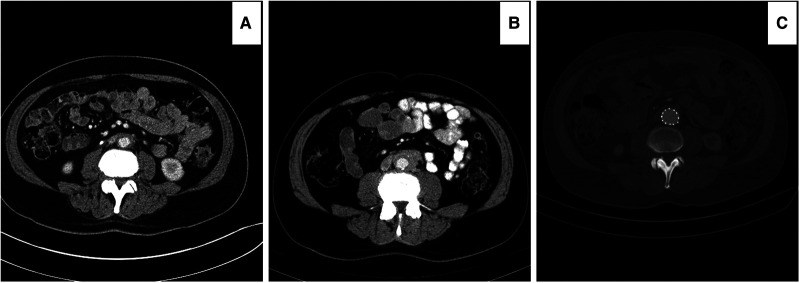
Computed tomography angiography (CTA) images showing improvement in peri-aortic soft tissue enhancement following endovascular aneurysm repair (EVAR): (A) peri-aortic enhancement of 3 mm on optimal medical therapy for three months. (B) Peri-aortic enhancements increased to 6 mm after optimal medical management for nine months. (C) peri-aortic enhancements disappeared with no evidence of RPF six months post-EVAR.
